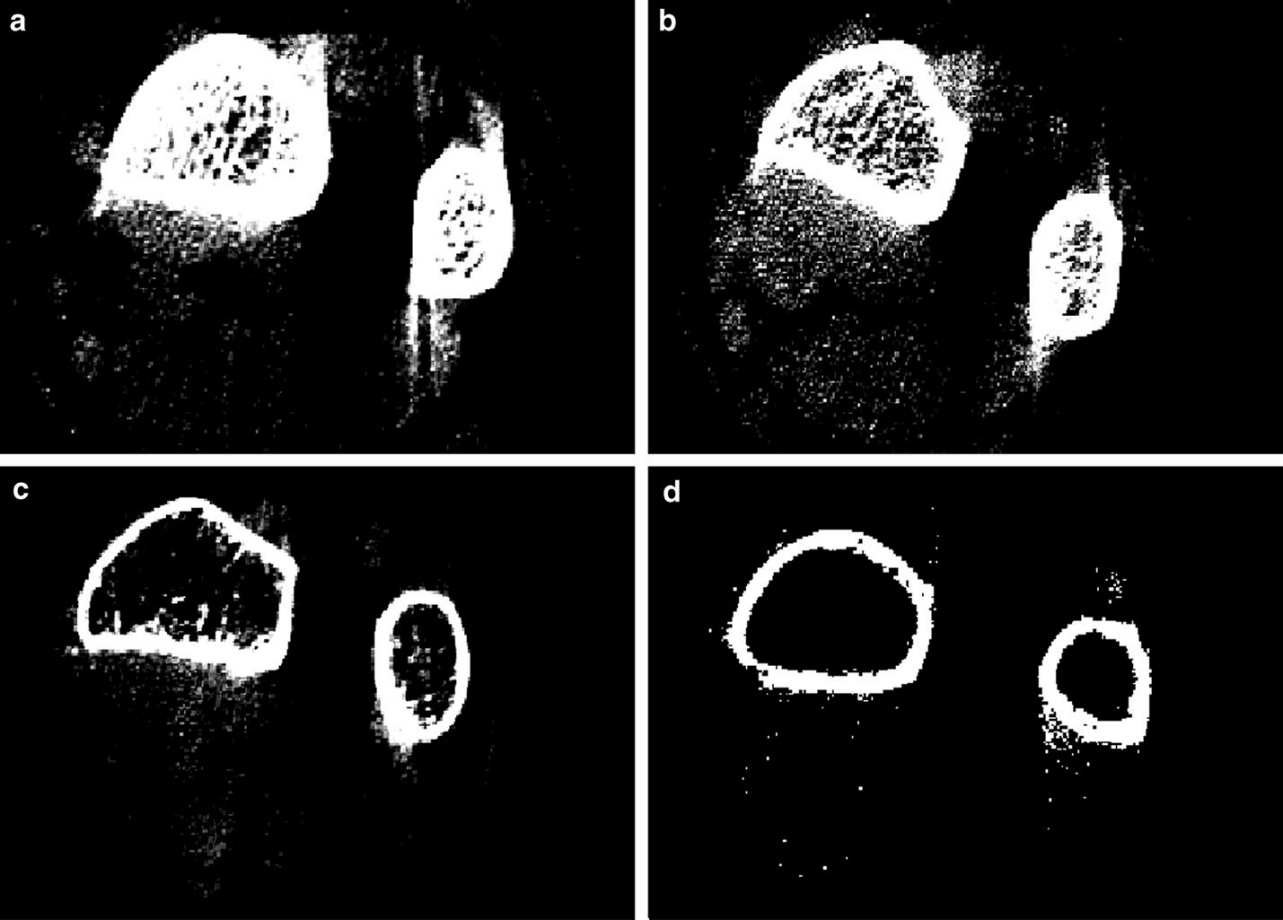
Scanned forearm CBCT images; a, b From two healthy subjects, c, d From osteoporosis patients
